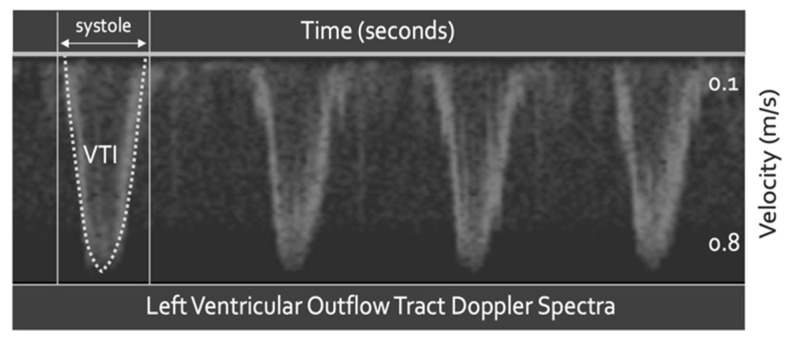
Doppler ultrasound of the left ventricular outflow tract; 4 cardiac cycles obtained via transesophageal echocardiography. The dotted trace is the maximal velocity and the area under that trace is the velocity time integral (VTI).
